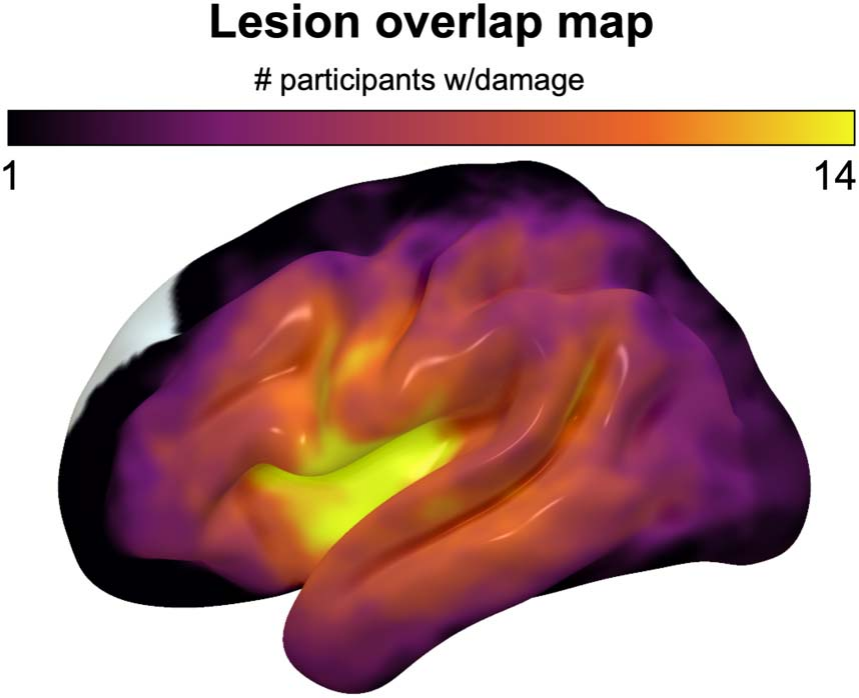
Lesion overlap map for participants with aphasia. Map represents all aphasia participants (*n* = 25), without one participant for whom MRI was contraindicated, with a maximum overlap of 14 individuals in a given region.
